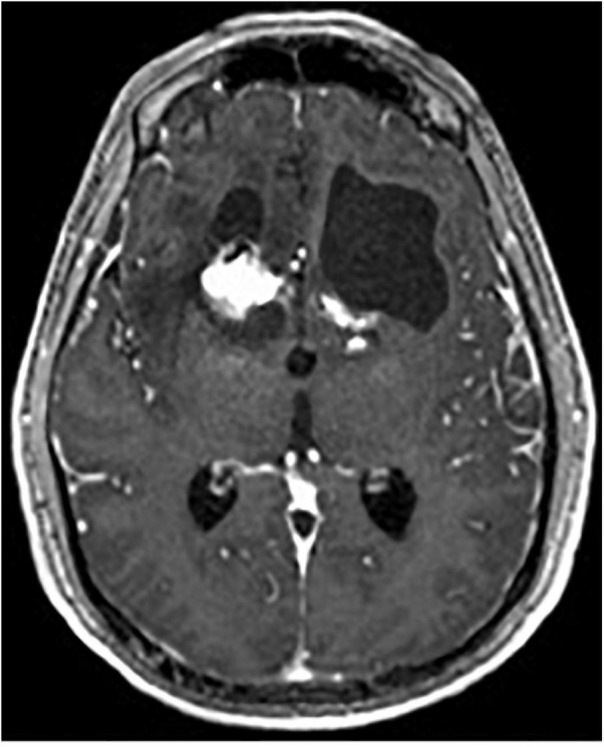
Axial T1-weighted MRI with contrast of brain cyst in the left hemisphere after gamma knife capsulotomy.
